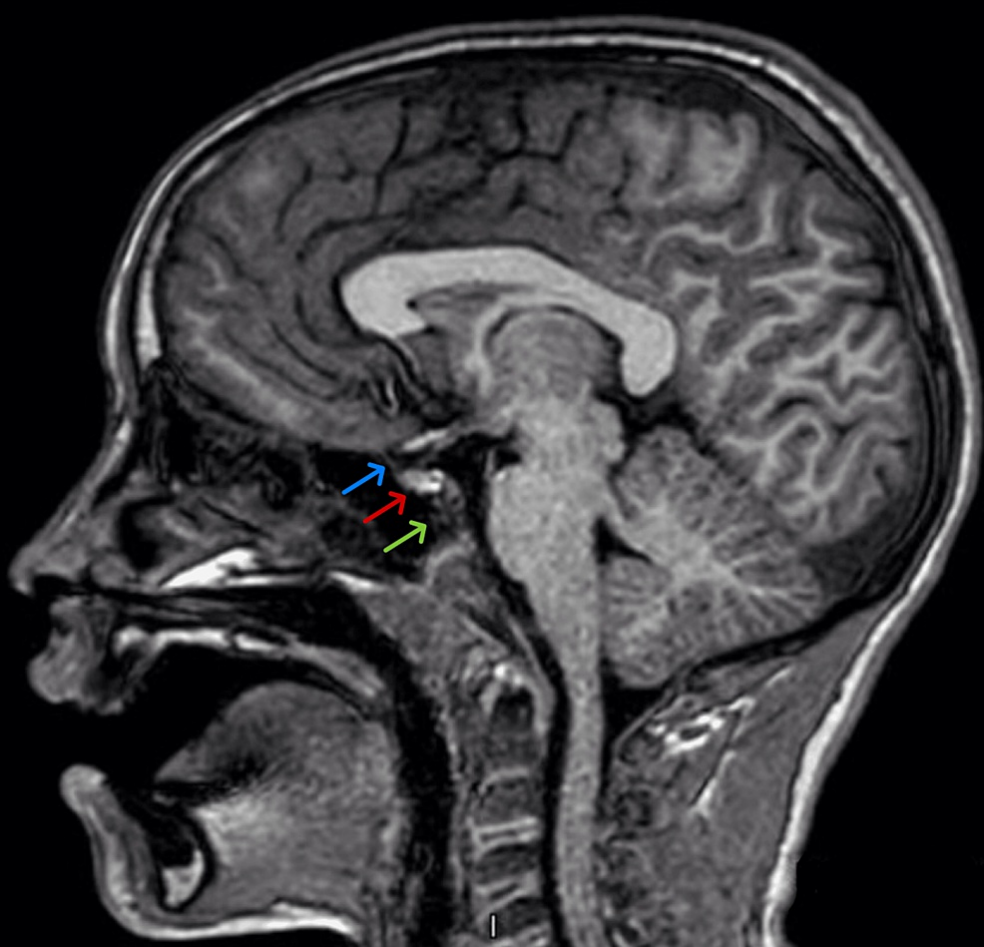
Sagittal T1 Brain MRI Magnetic resonance imaging shows no brain pathology and a normal pituitary stalk (blue arrow), anterior pituitary (red arrow), and posterior pituitary (green arrow).
